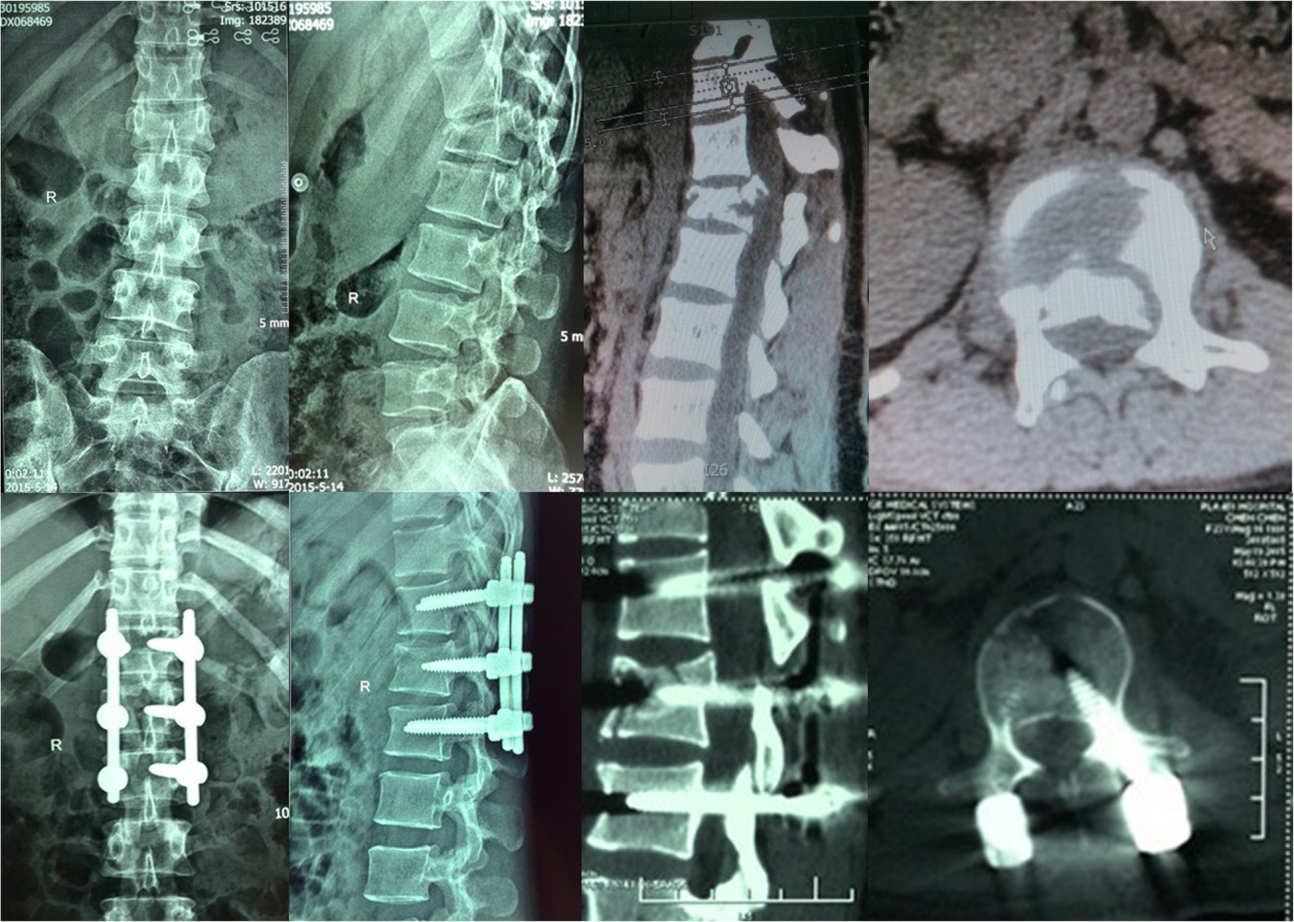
A 23 years old female with L1 burst fracture treated by the present new approach surgery. Preoperative and postoperative X-ray and CT are shown. The canal encroachment were efficiently relieved, as well as the sagittal kyphosis angle and percentage of anterior height of the fractured vertebra were all significantly improved postoperatively
